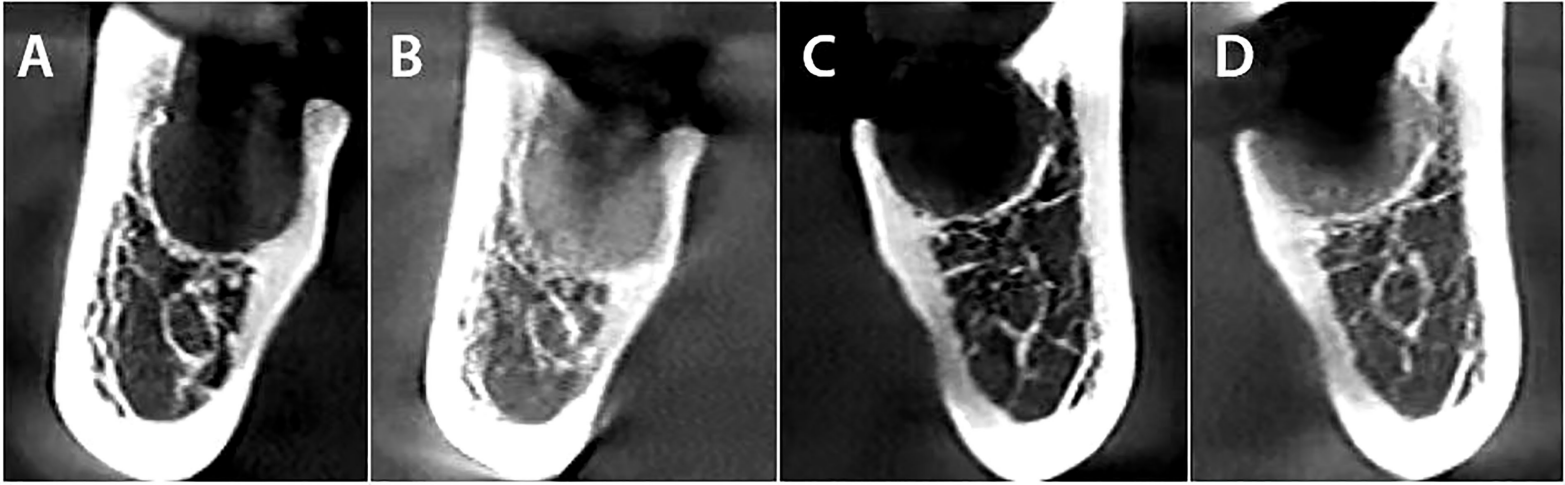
The comparison between both sides immediately after extraction, followed by a CBCT three months later. (A) CGF side immediately after extraction. (B) CGF side three months after the dental extraction. (C) Control side immediately after extraction. (D) Control side three months after the dental extraction.
